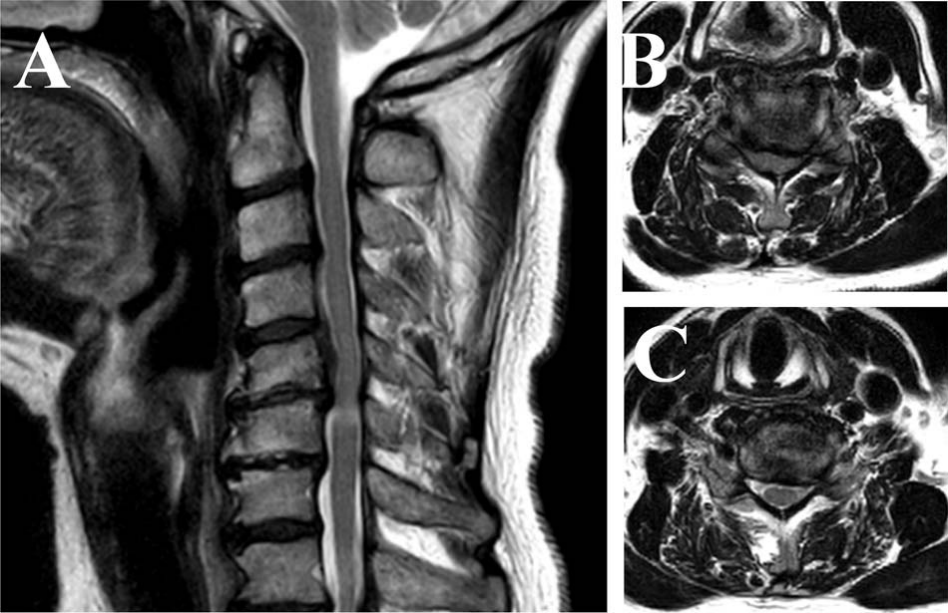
Magnetic resonance imaging of the cervical spine (T2-weighted image). (A) Sagittal view images showed that compressive lesions in the cervical spine were located at the C5-C6 levels. The high signal lesion in the spinal cord was seen at the C5-C6 level. (B) Axial view of the C5-C6 level showed the spinal cord compression in the canal. (C) Axial view of the C6-C7 level showed the stenosis of the left side of foramen.
